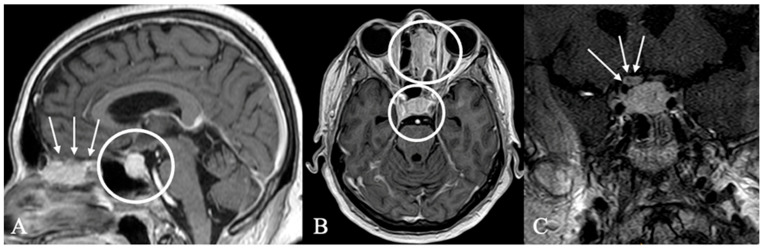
A 60-year-old female patient with a diagnosis of granulomatous inflammation. (A,B) Sagittal and axial contrast-enhanced MRIs show a contrast-enhancing mass in the sellar and paranasal sinuses (circle, arrows). (C) Coronal FLAIR MRI shows a contrast enhancement of the right optic nerve. Lumbar puncture revealed signs of an inflammatory process. Intraoperatively, the lesion showed yellowish soft granules that were easy to dissect and biopsy. There was no relevant vasculature or bleeding risk.
